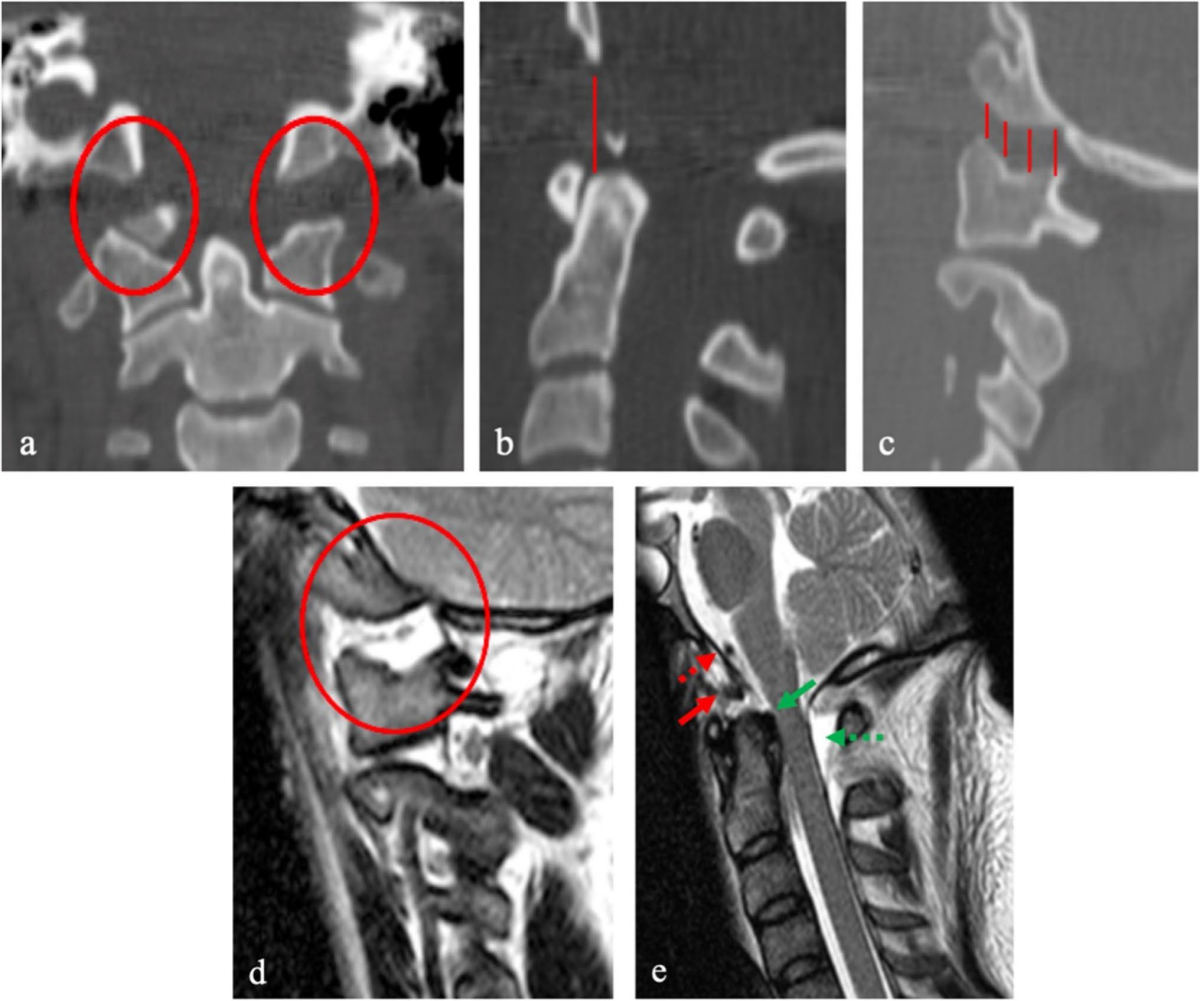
Atlantoaxial dislocation type II. a CT scan, coronal view, showing an increased occipital condyle-C1 interval (red circles). The condylar sum is defined as the sum of a patient’s left and right mean cranio-cervical interval (CCI) values. A distance > 2 mm in adults or > 5 mm in children, or a gross asymmetry between the two C0–C1 joints is highly sensitive and specific for AOD. b CT scan, sagittal view, focusing on basion-dens interval (red line). c CT scan, sagittal view, displaying an increased occipital condyle-C1 interval (red lines). d T2-weighted MRI, sagittal view, pointing out a fluid signal in the atlanto-occipital joint (red circle). e T2-weighted MRI, sagittal view, showing abnormal signal at the basion-dens interval, translating the disruption of the anterior atlanto-occipital membrane (red arrow), apical ligament (dotted red arrow), tectorial membrane (green arrow), and posterior atlantooccipital membrane (green dotted arrow)
